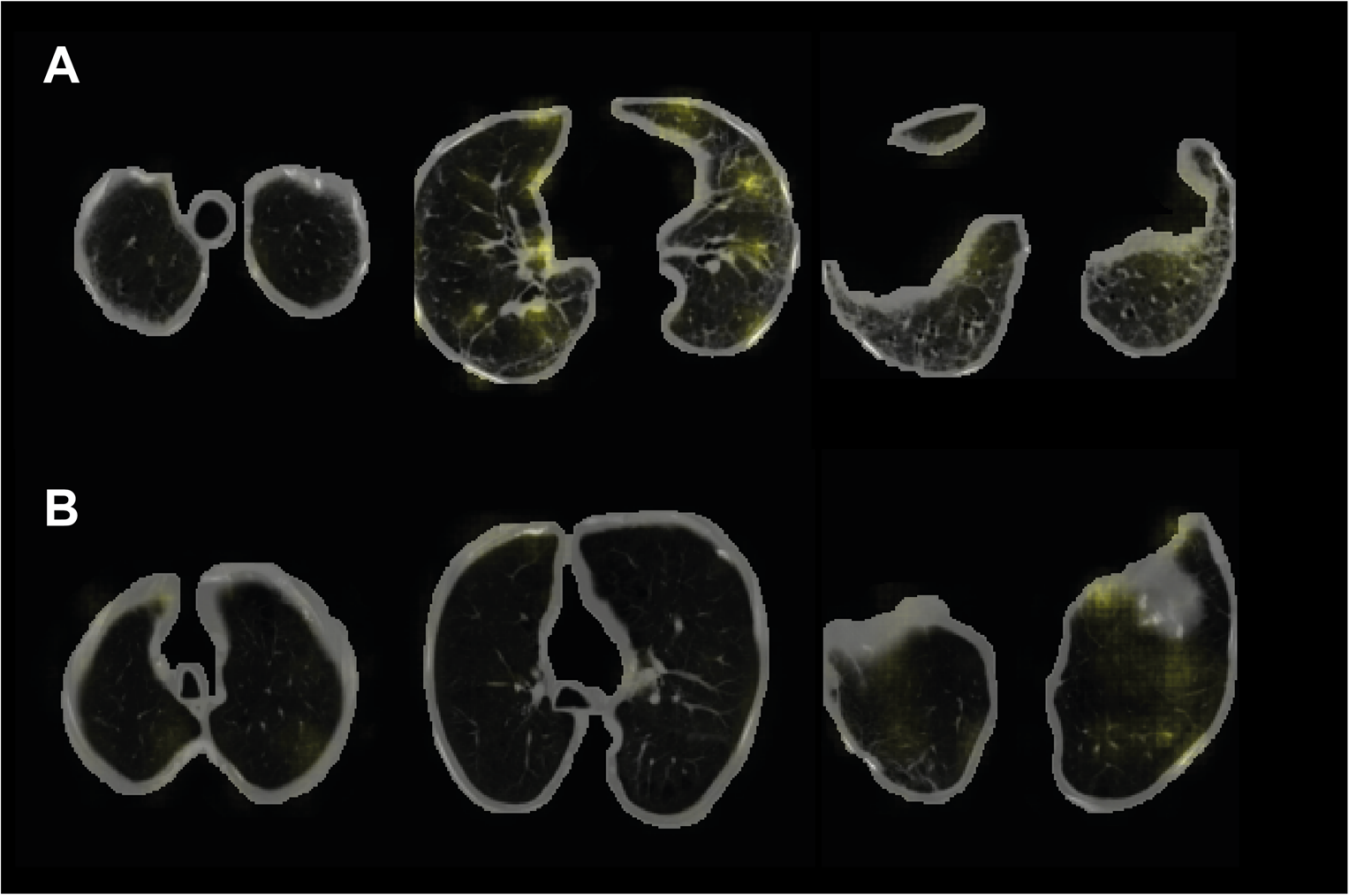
Saliency map yielded by the AI model of two CT scans in the test set. The areas in yellow represent the attention of the model. The left side shows a slice from the top of the lungs, the middle a slice in the middle of the lungs, and the right side a slice from the bottom of the lungs. A CT scan where 3/3 pulmonologists were positive and the model yielded a high probability of asbestosis (0.81). B CT scan where 0/3 pulmonologists were positive and the model yielded a low probability of asbestosis (0.19)
